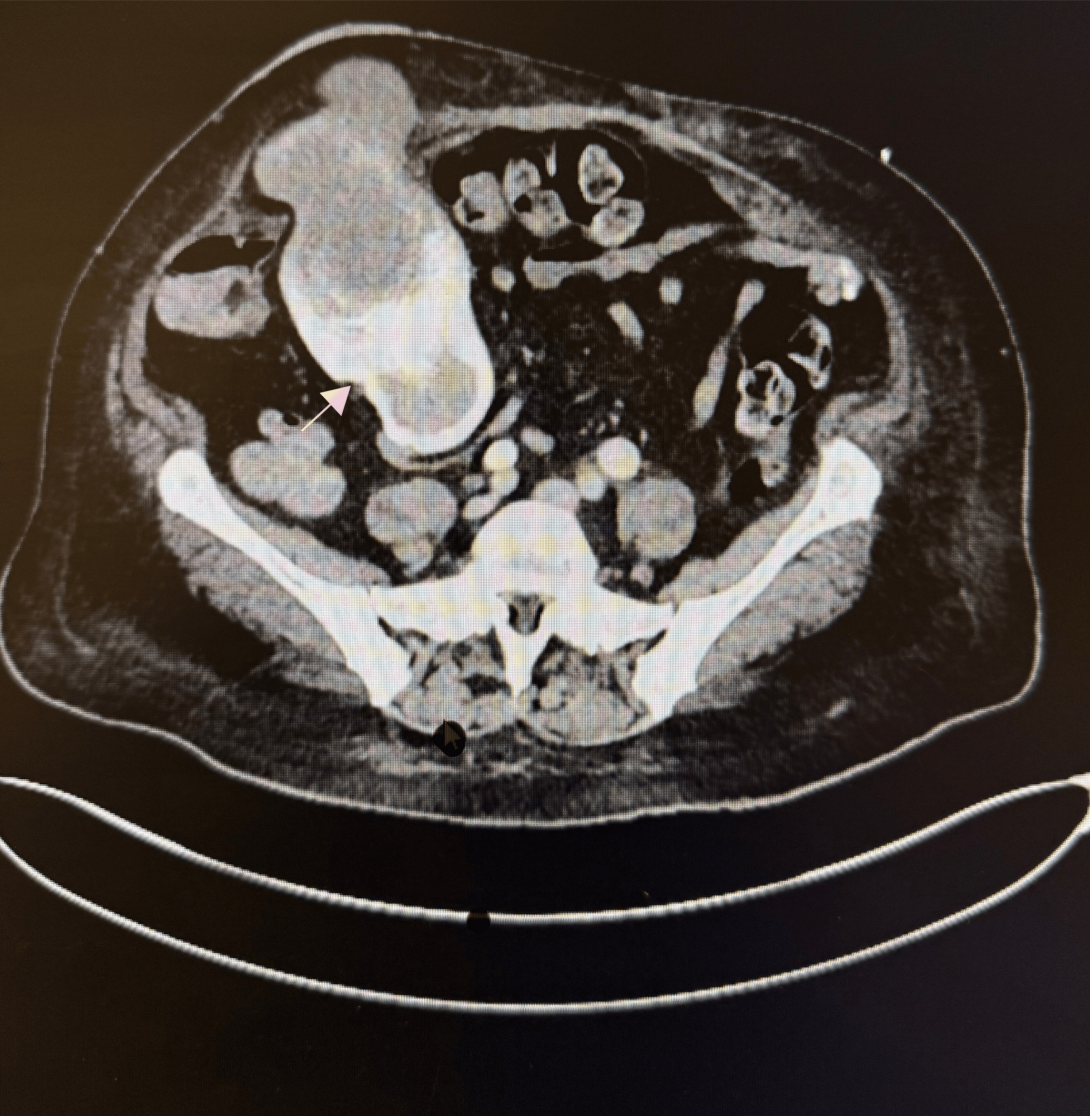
CT angiography confirmed active contrast extravasation within the ileal conduit.
